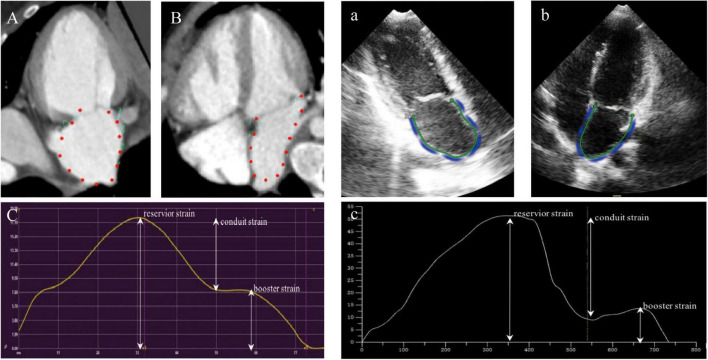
Example for the assessment of LA strain using cardiac computed tomography (CCT) and TTE in two-chamber view (A,a), three-chamber view (B,b), and the strain curve of the result (C,c).
